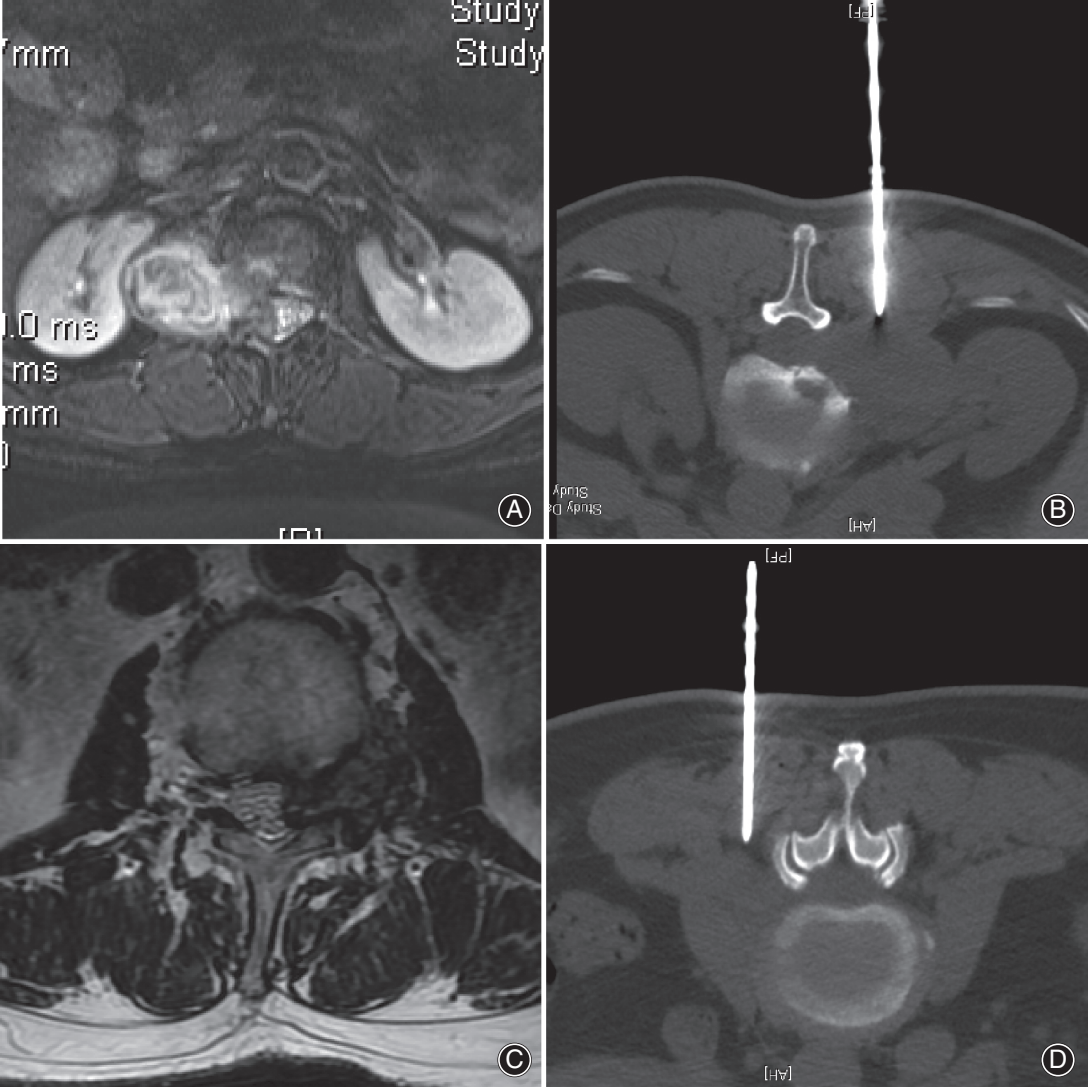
(A) MRI showed a paravertebral soft tumor in a 61‐year‐old woman. (B) A diagnosis of schwannoma was given by CT‐guided biopsy. (C) MRI showed a soft tumor located in the intervertebral foramina in a 76‐year‐old man where it was difficult to guide by C‐arm only. (D) CT‐guided biopsy revealed it was a metastasis of renal cell carcinoma.
